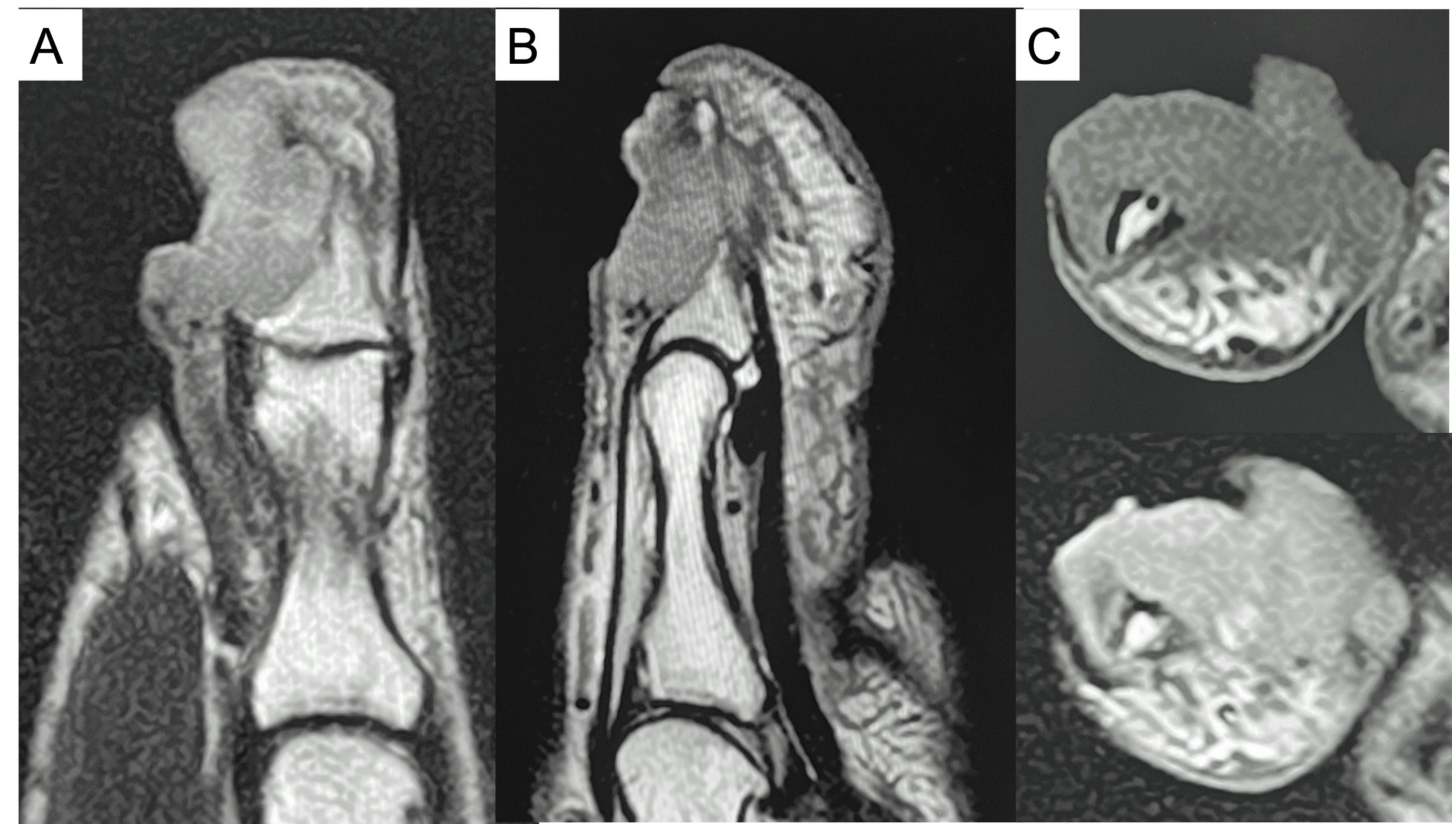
MRI images (A: coronal view of the T2-weighted image, B: sagittal view of the T2-weighted image, C: axial view (top: T1-weighted, bottom: T2-weighted).
